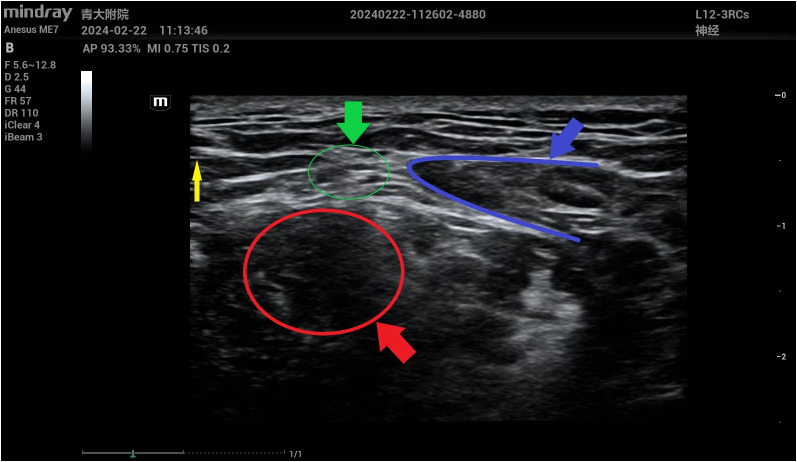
Anatomic marker in ultrasound images of superficial cervical nerve anesthesia under ultrasound guidance. The yellow arrow indicates the puncture needle; The green arrow indicates the superficial cervical plexus; The selected area in the blue frame represents the lateral margin of the sternocleidomastoid muscle; The area selected in the red wire frame indicates the middle scalene muscle.
